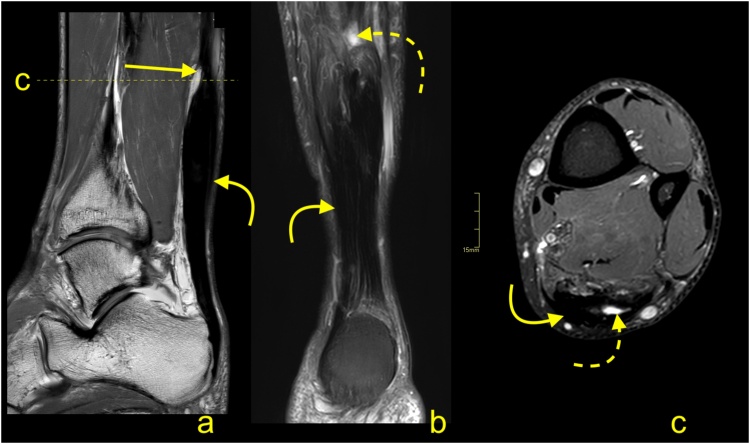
Chronic Achilles tendon rupture with some dynamic inefficient. A 58-year-old patient who suffered a ruptured Achilles tendon 1 year ago. Clinical suspicion of tendon elongation. The MRI (a–c) revealed a thickened and relaxed Achilles tendon, which is a sign of elongation. In the proximal part of the tendon, a posttraumatic substance defect in the tendon is visible (straight arrow), and in the midportion, thickening of the tendon is present (curved arrow). In the defect, a small amount of fluid is visible (dashed curved arrow). A- PD- weighted, b- T2- weighted with fat suppression, c- PD- weighted with fat suppression.
